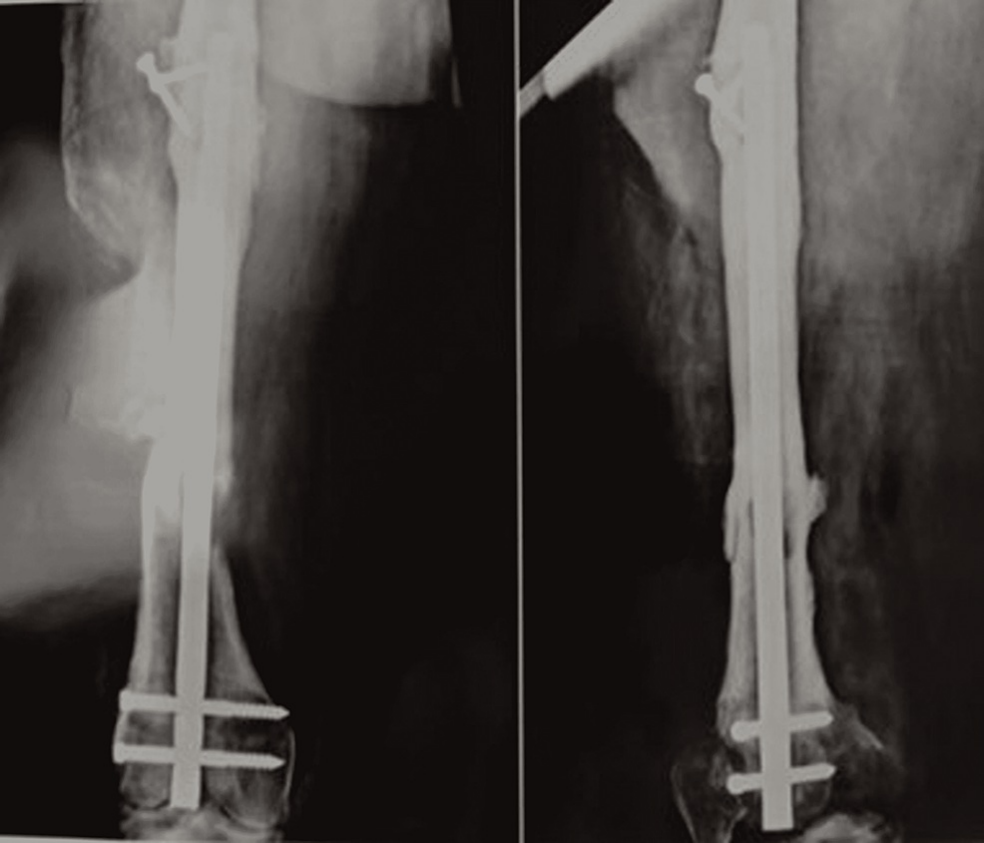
X-ray showing union with the femoral nail in situ.
